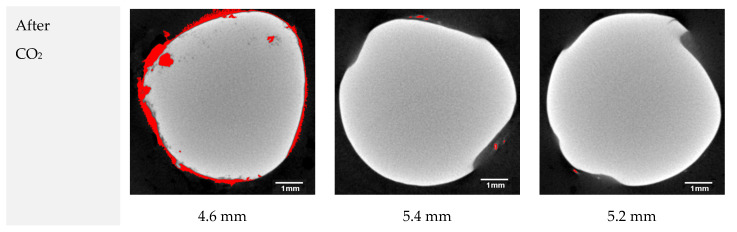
Micro X-ray computer tomography (µXCT) images of steel reinforcement cross sections in the mortar samples with the smallest mortar cover (see values). The red area shows new reaction products with a lower density than non-corroding steel in the transition zone between steel and mortar matrix after leaching and accelerated carbonation (*CO_2_* chamber).
